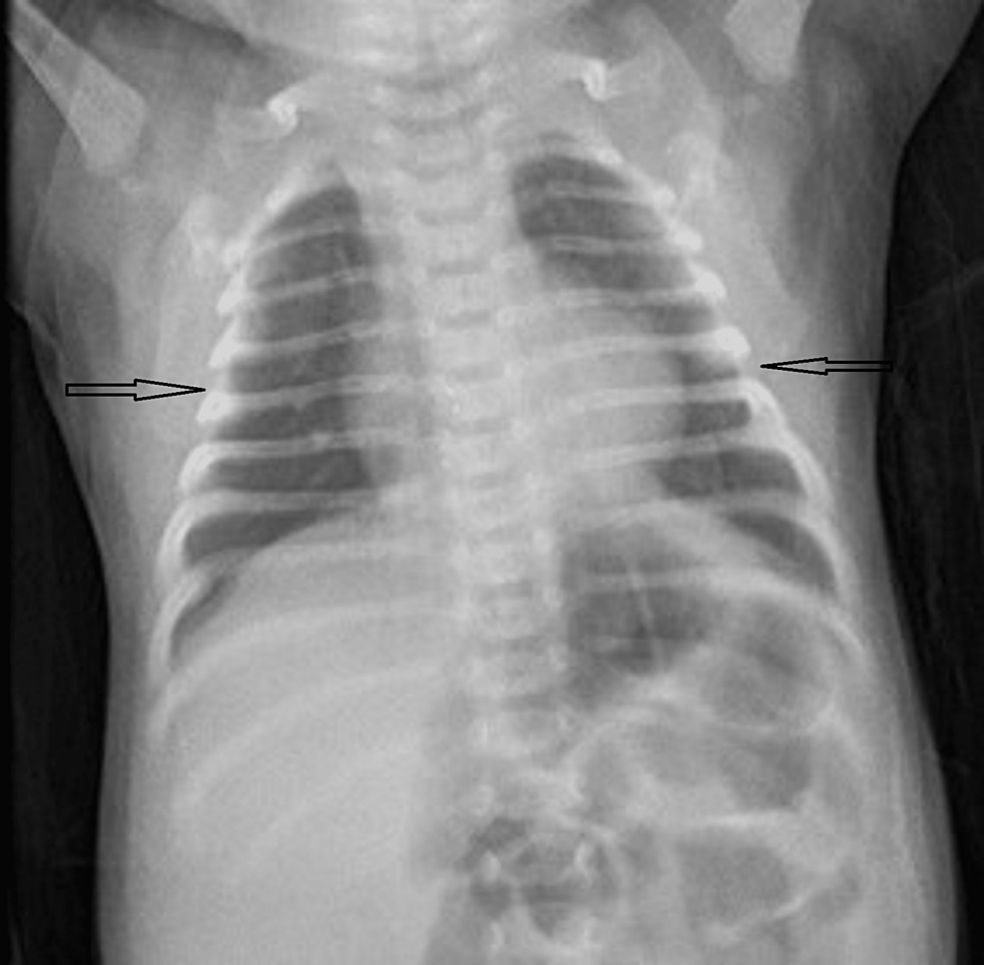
X-ray of the chest showing bilateral clear lung fields.
